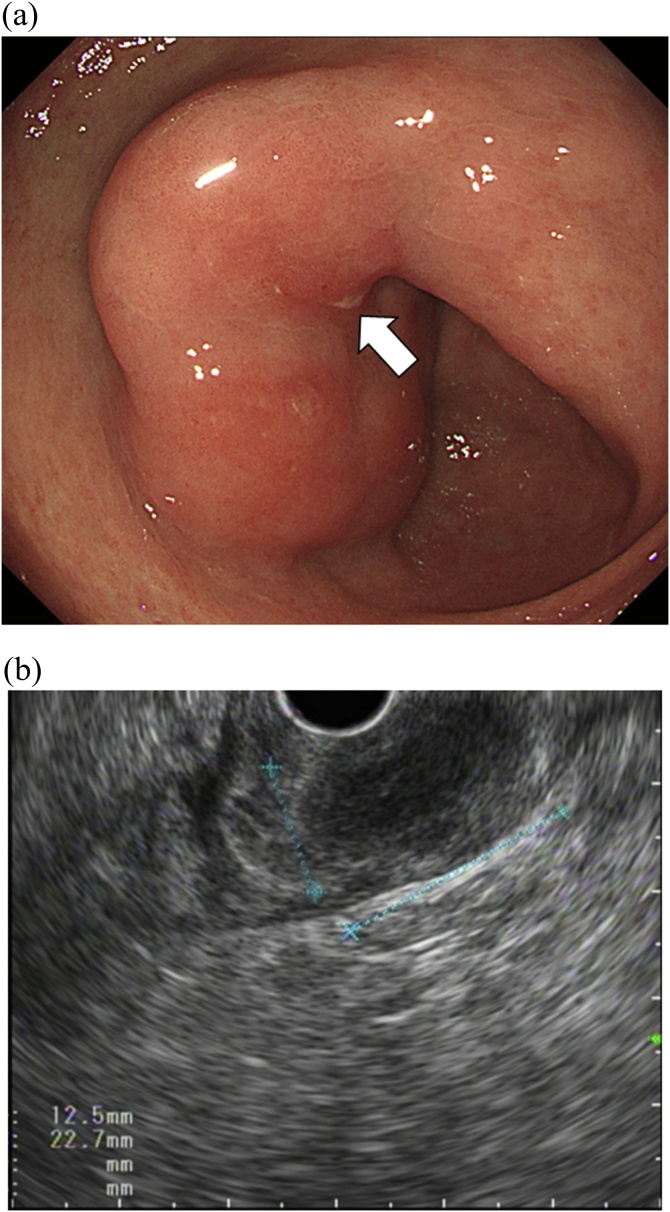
Preoperative endoscopic examination and endoscopic ultrasonography. (A) Endoscopic image of the submucosal tumor. The surface of the lesion is covered by non-malignant mucosa with erosion (arrow). (B) Endoscopic ultrasound examination showed a well-defined hypoechoic mass (22 × 12 mm), which originates from the submucosal layer of the stomach.
